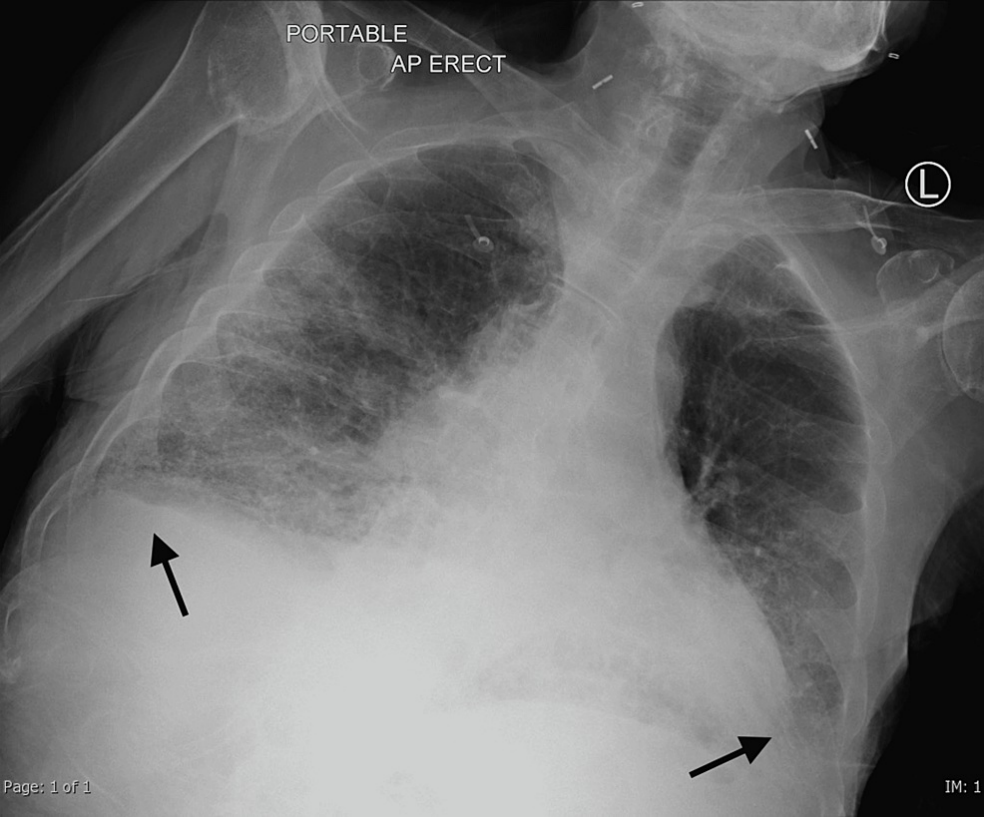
Chest X-ray showing extensive bilateral air space infiltration and small bilateral pleural effusions (arrows)
